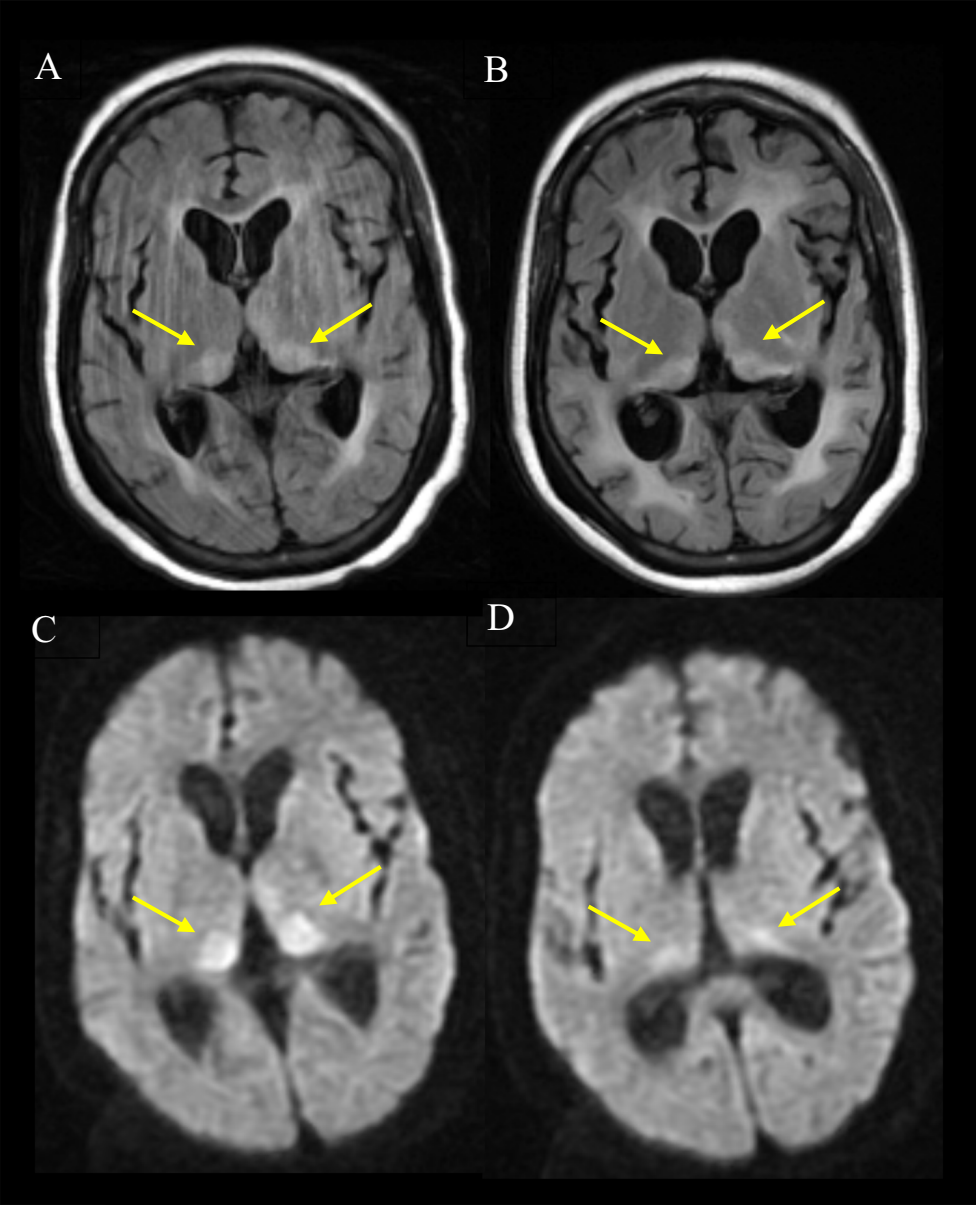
Serial MRI scans (A+C) and follow up scan at 6 months (B+D) of neuropathologically confirmed case of Alzheimer’s disease with positive pulvinar sign. Axial FLAIR sequences (A+B) showing positive pulvinar sign (A) (arrows) with (B) associated dorsomedial thalamic high signal on repeat scan (arrows). DWI sequences (C+D) demonstrating more conspicuous pulvinar high signal (arrows) with (D) less marked thalamic hyperintensity on follow up scan (arrows)
